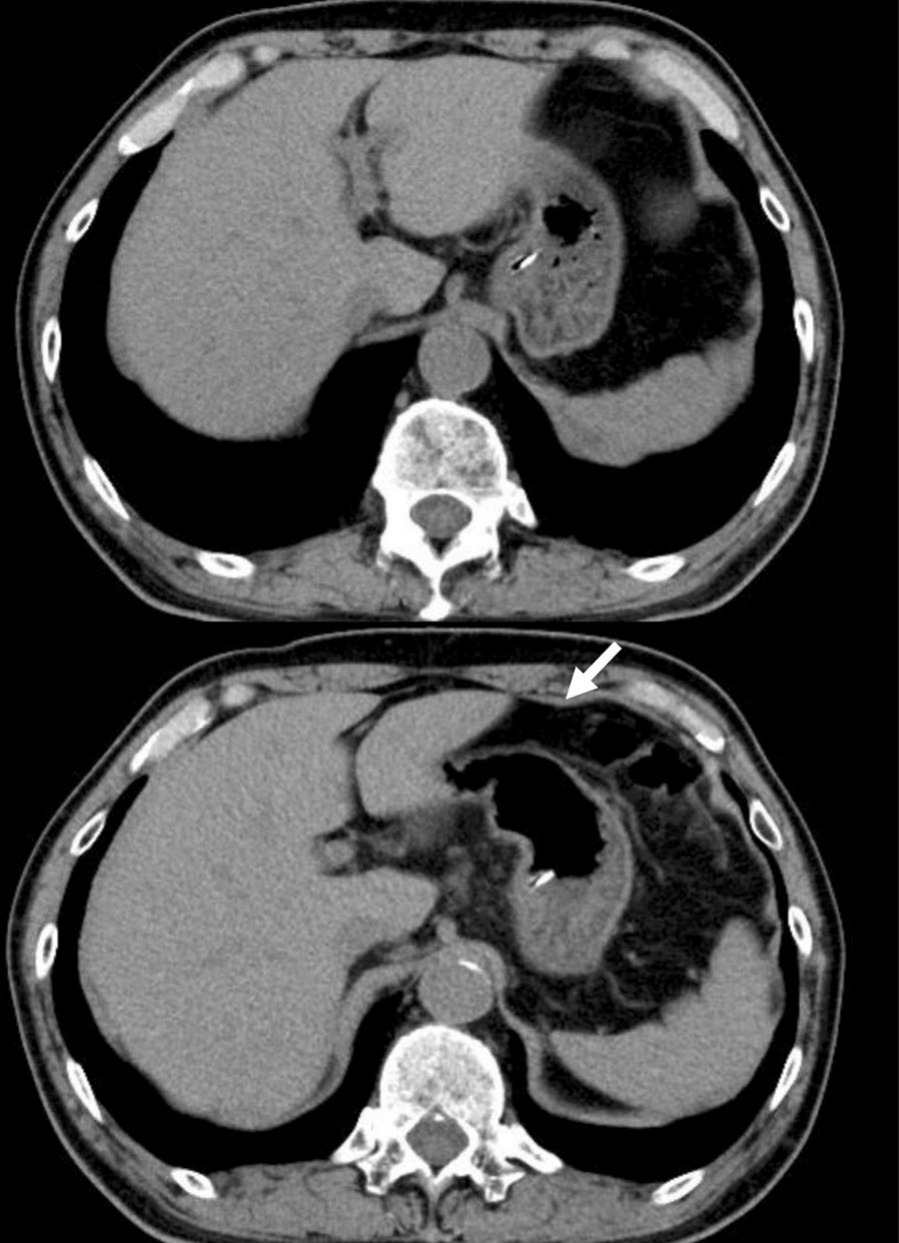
Computed tomography revealed the left lateral segment of the liver and transverse colon (arrow) between the remnant stomach and abdominal wall
